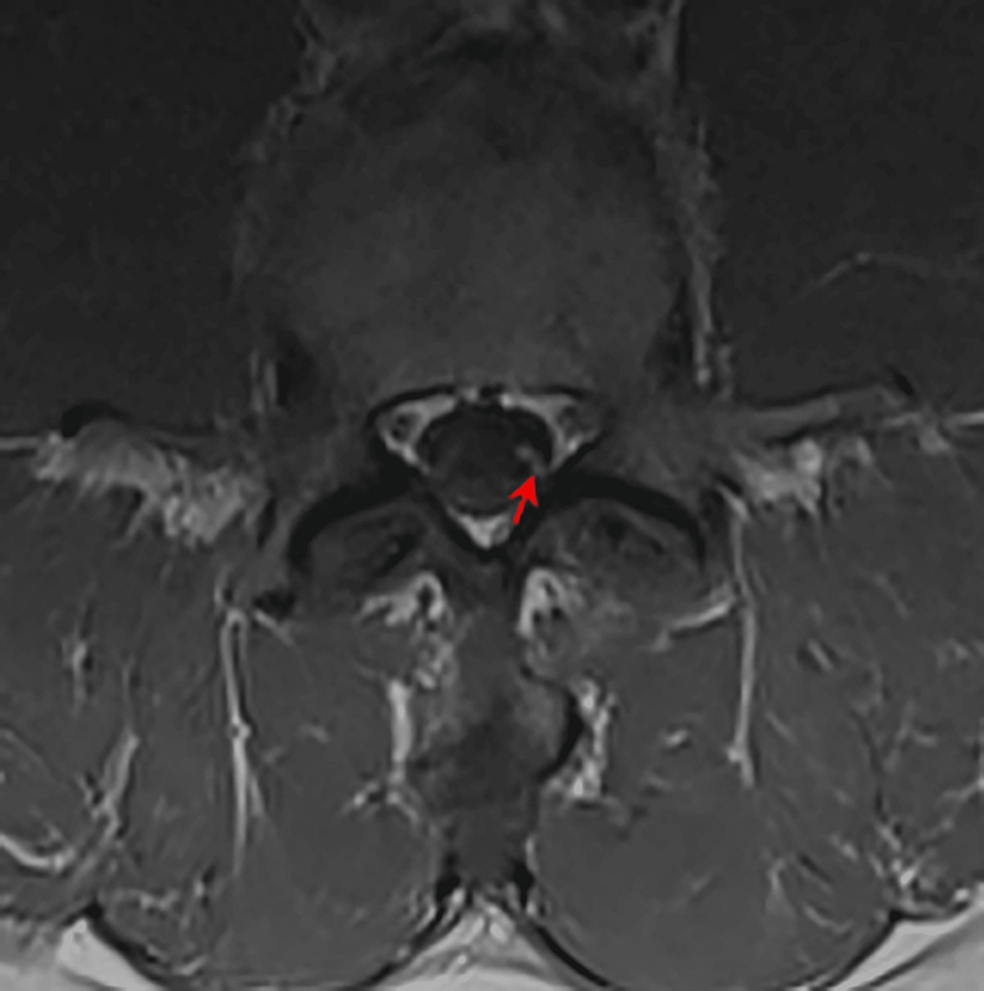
MRI lumbar spine image Arrow: enhanced left L5 nerve root
